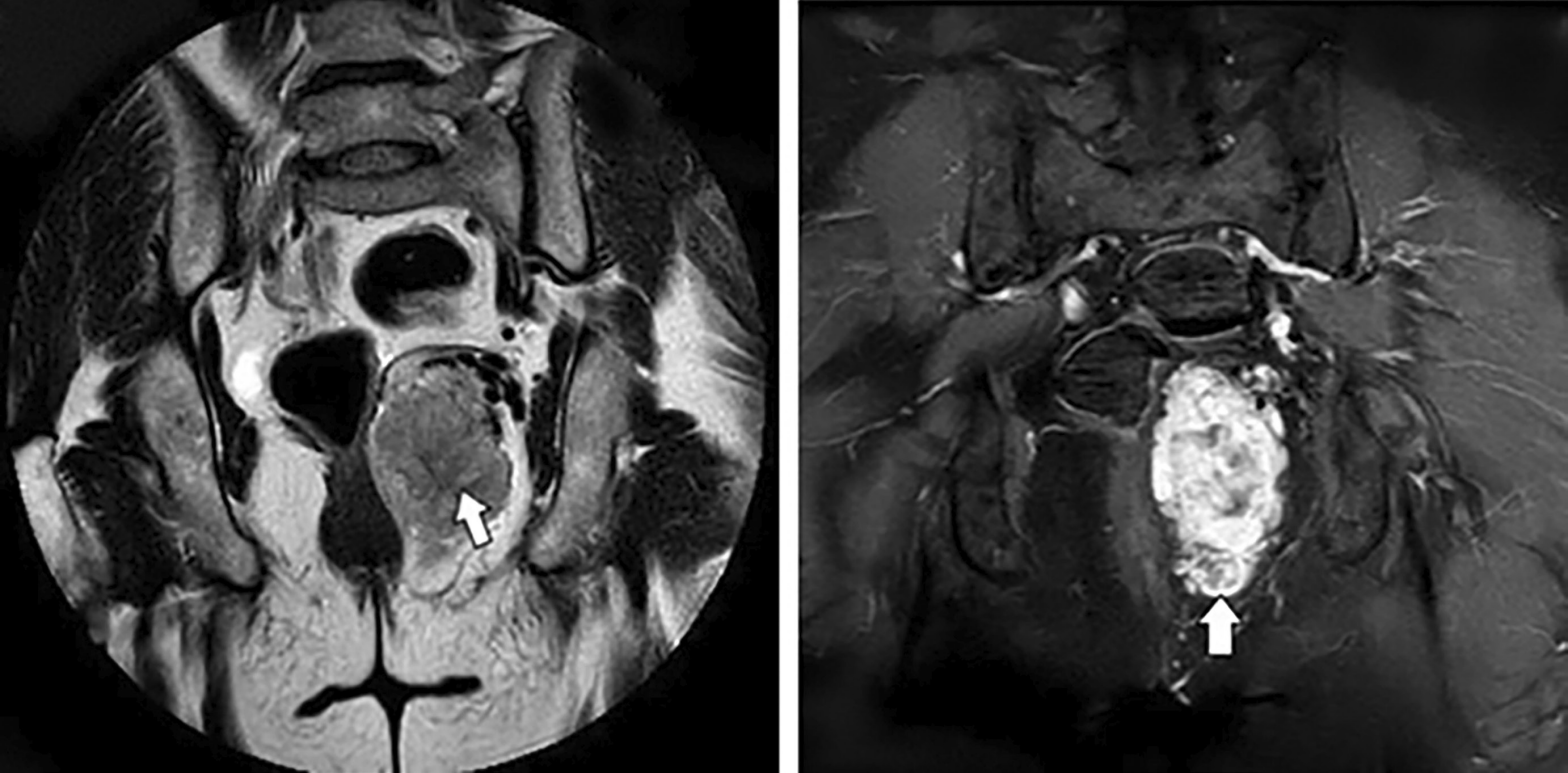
Pelvic MRI. Arrows indicate a large enhancing T2 signal intensity lesion in the left side of peri‐anal soft tissue.
